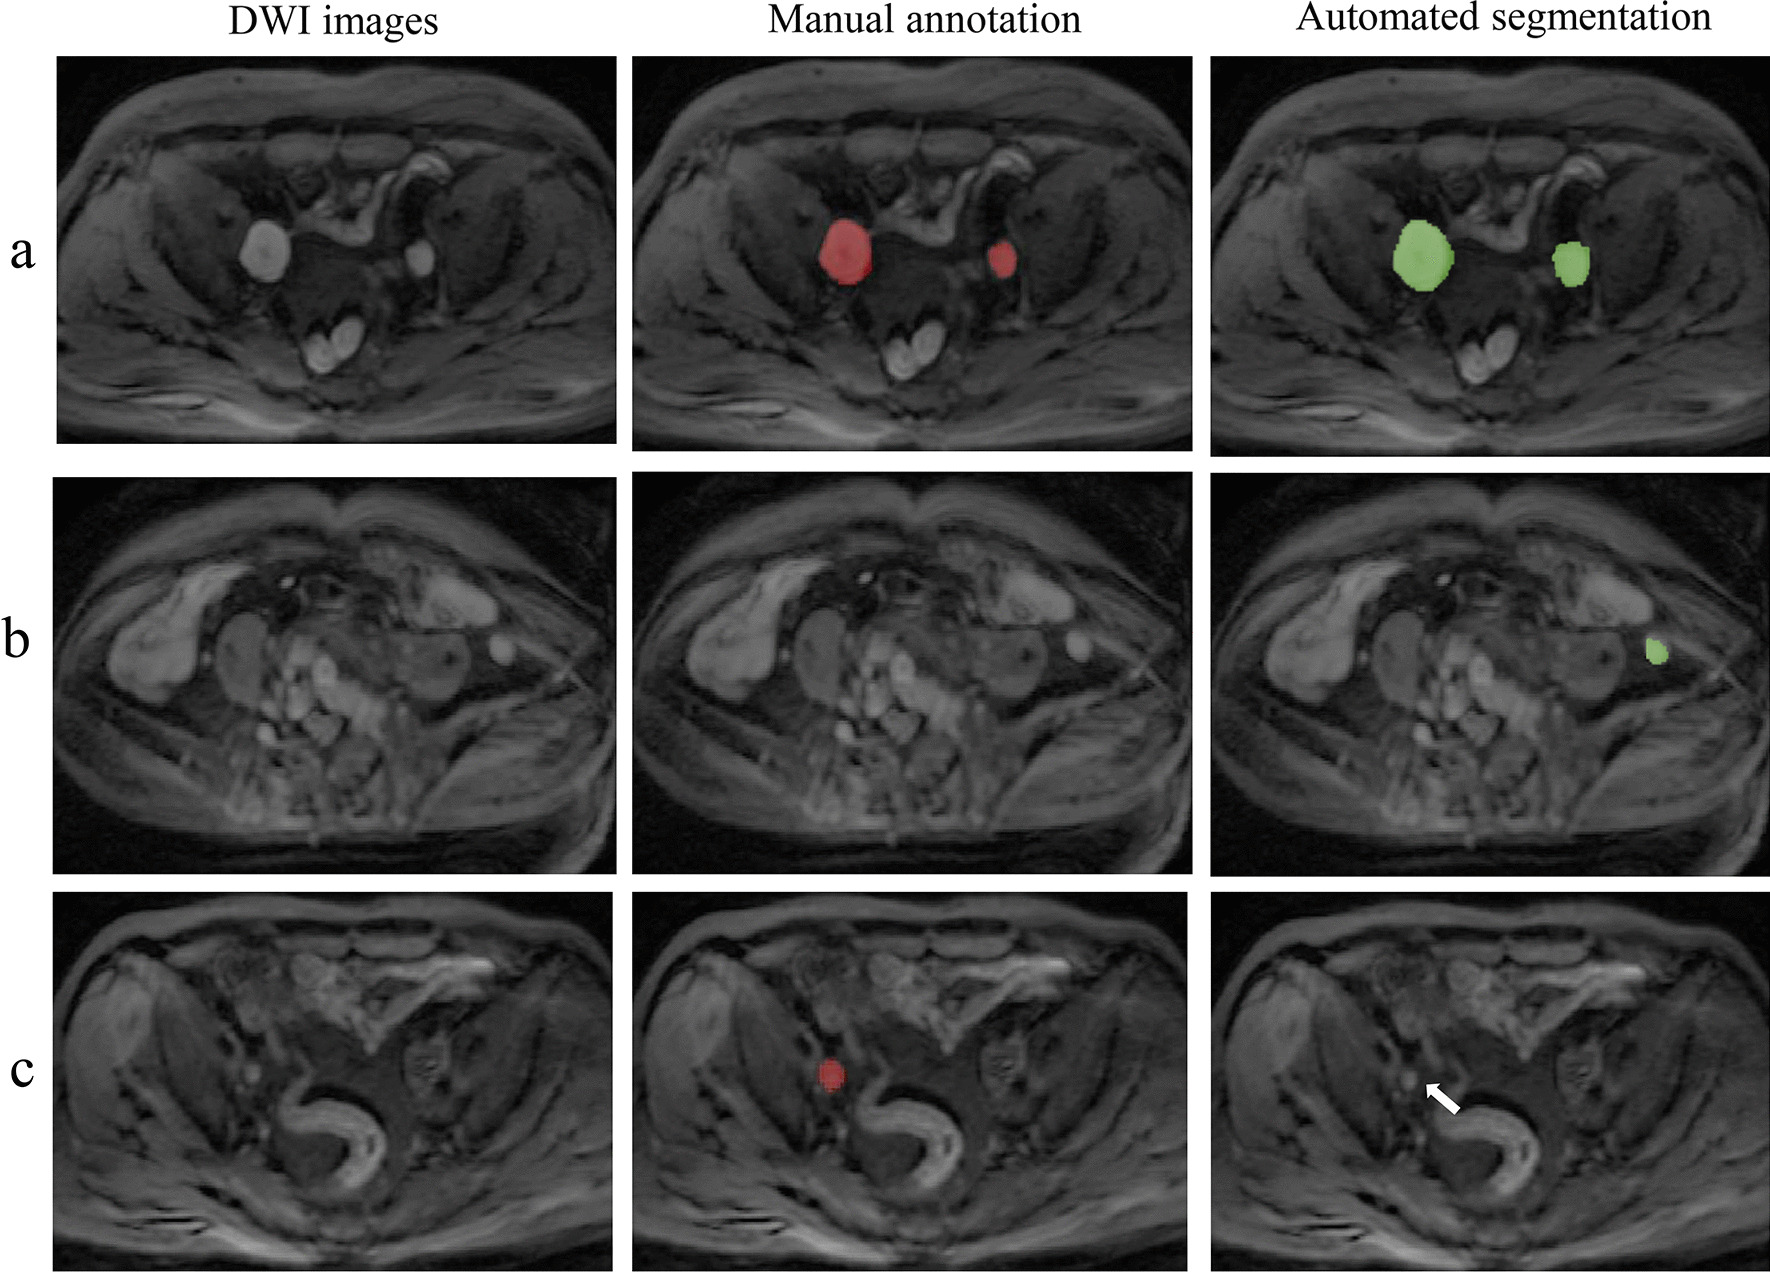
Examples of the detection results of the 3D U-Net for the lymph nodes. The first column represents the DWI images; the second column represents the manual annotations, and the third column represents the automated segmentations. a The true positive LNs detected by the model (Green label in automated segmentation); b The false positive detection that was a part of the colon (Green label in automated segmentation); c The false negative detection that was missed by the model (Arrow points in the automated segmentation)
